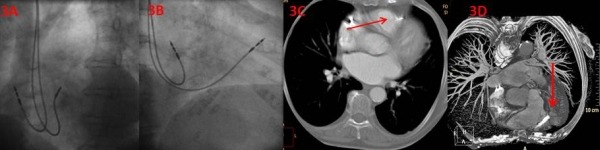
A. Appearance of the right ventricular lead in the LAO40 view. The original fluoroscopic position from LAO 40 view; B. Appearance of the right ventricular lead in the RAO30 view. The original fluoroscopic position from RAO 30 view; C. Cardiac CT showing the actual location of the tip of the lead in the anterior wall (anteroseptal groove); D. Spatial relationship and close proximity of the tip of the RV lead to the left anterior descending artery.
